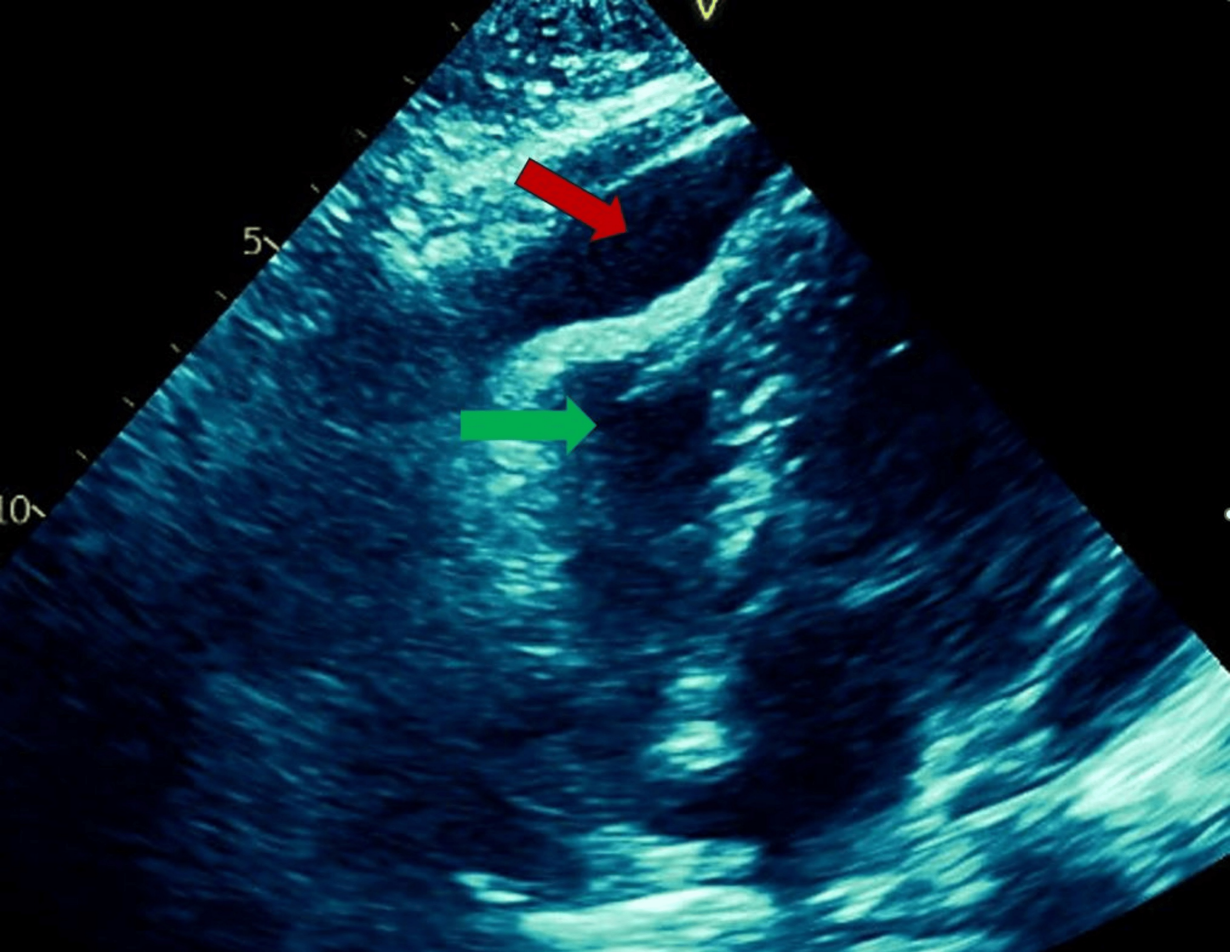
Transthoracic echocardiogram with red arrow demonstrating pericardial effusion causing the right ventricle to collapse (green arrow).
